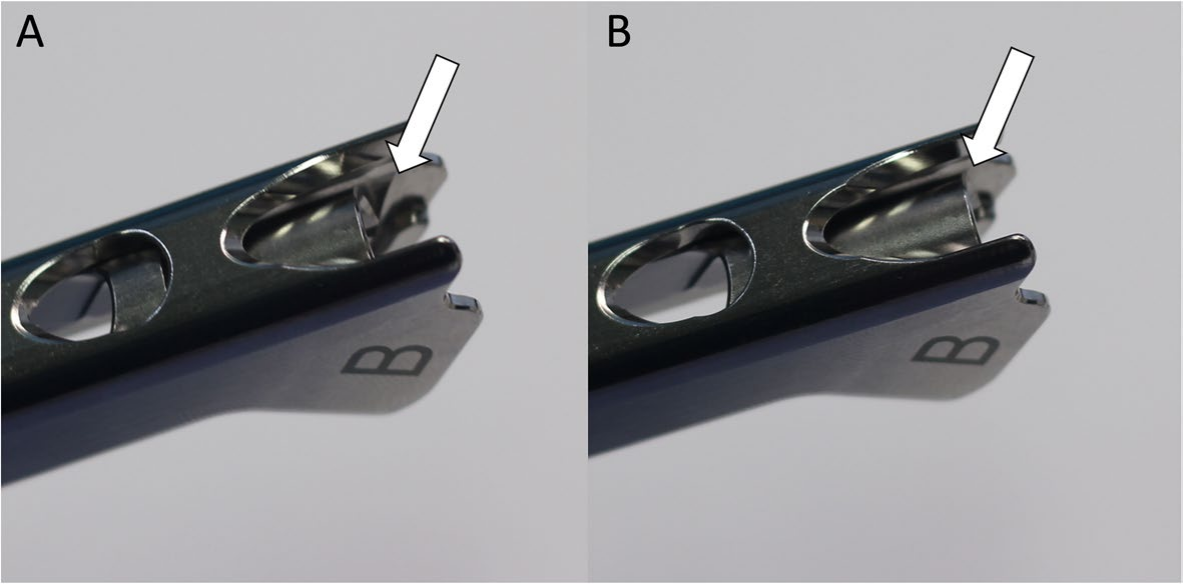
The radiographs show the connection between plate of FNS and insertion handle by tightening of black screw. A The white arrow indicates the slit for connecting with plate. If the black screw is completely loosened with insertion hand, plate can be disconnected from insertion handle. B The white arrow means that plate slit is blocked by metal portion of black screw. If the black screw is tightened even a little, plate cannot be disconnected from handle. The photographs were taken by the authors
